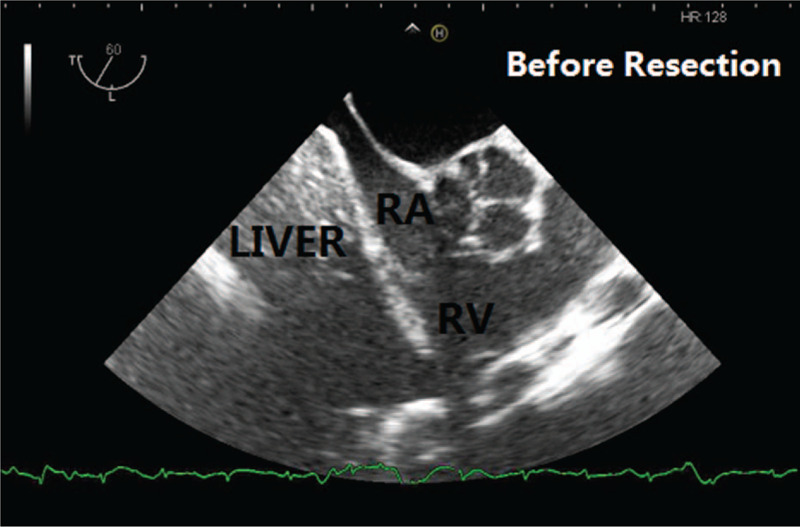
TEE image (ME RV inflow-outflow Tract) of the first patient before resection. The patient's diaphragm and liver were lifted due to compression and the right atrium's filling was limited. TEE = transesophageal echocardiography.
